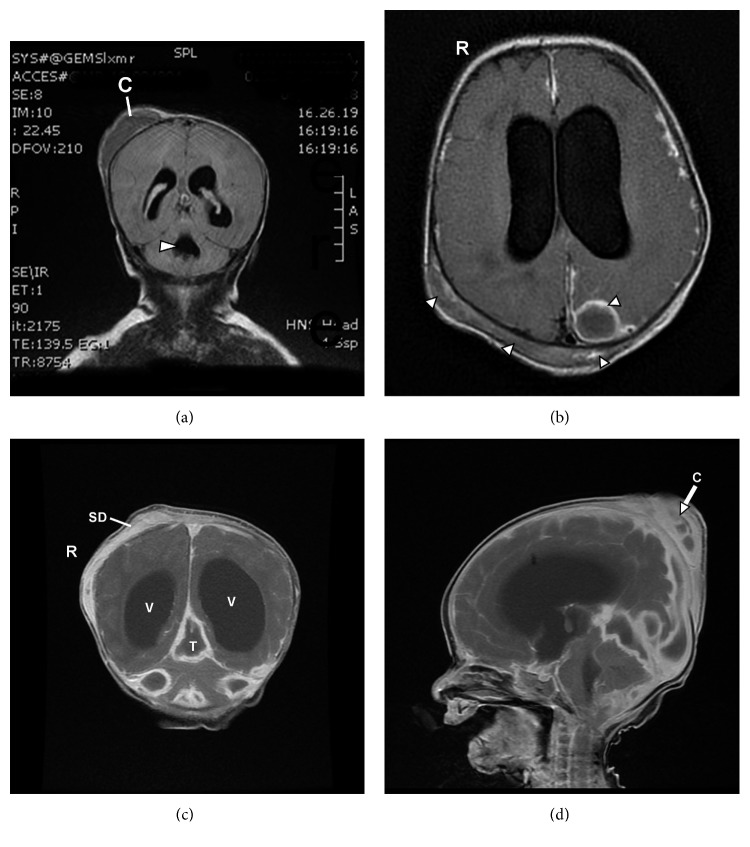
(a) MRI of the head performed on the second hospital day showing mildly dilated lateral and fourth (arrow) ventricles. Cephalhematoma (C) was also noted. (b) Hospital day 13: MRI of the head showing moderately dilated lateral ventricles. Bilateral subdural and left parietooccipital parafalcine fluid collections were noted, suggestive findings of multiple abscesses. (c, d) Hospital day 19: MRI of the head showing multiple enhancing lesions in the bilateral posterior fossa, tentorium (T), and subdural (SD) region, suggestive findings of multiple brain abscesses and subdural empyema. Lateral ventricles (V) were moderately dilated.
